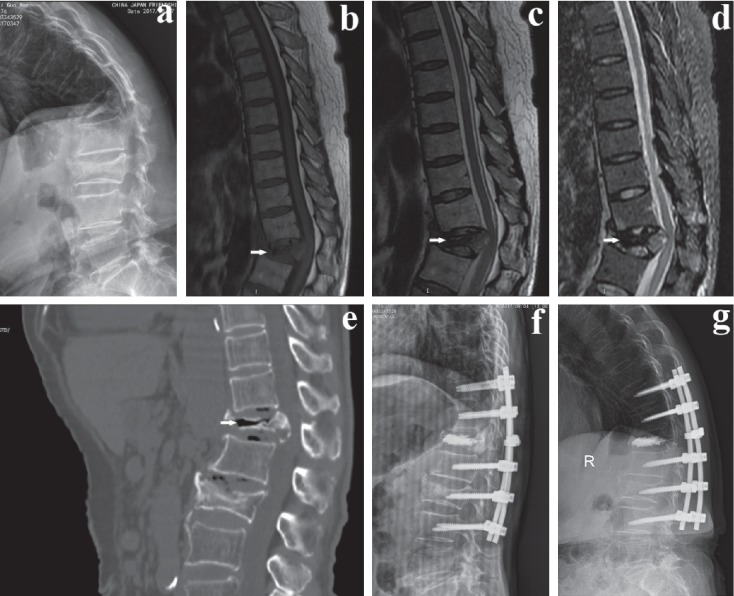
A 76-year-old male patient underwent PFVP because of L1 OVCFs with IVC complicated by neurological deficits. (a–e) Preoperative sagittal plain radiographs, T1- and T2-weighted MRI, STIR MRI, and CT showed an IVC and spinal cord compression at the L1 level with a collapsed fracture and kyphosis. (f) Immediate postoperative plain radiographs showed bone cement in the vertebral body with increased anterior vertebral height and resolved kyphosis. (g) Lateral radiographs showed kyphosis recurrence after 2 years.
